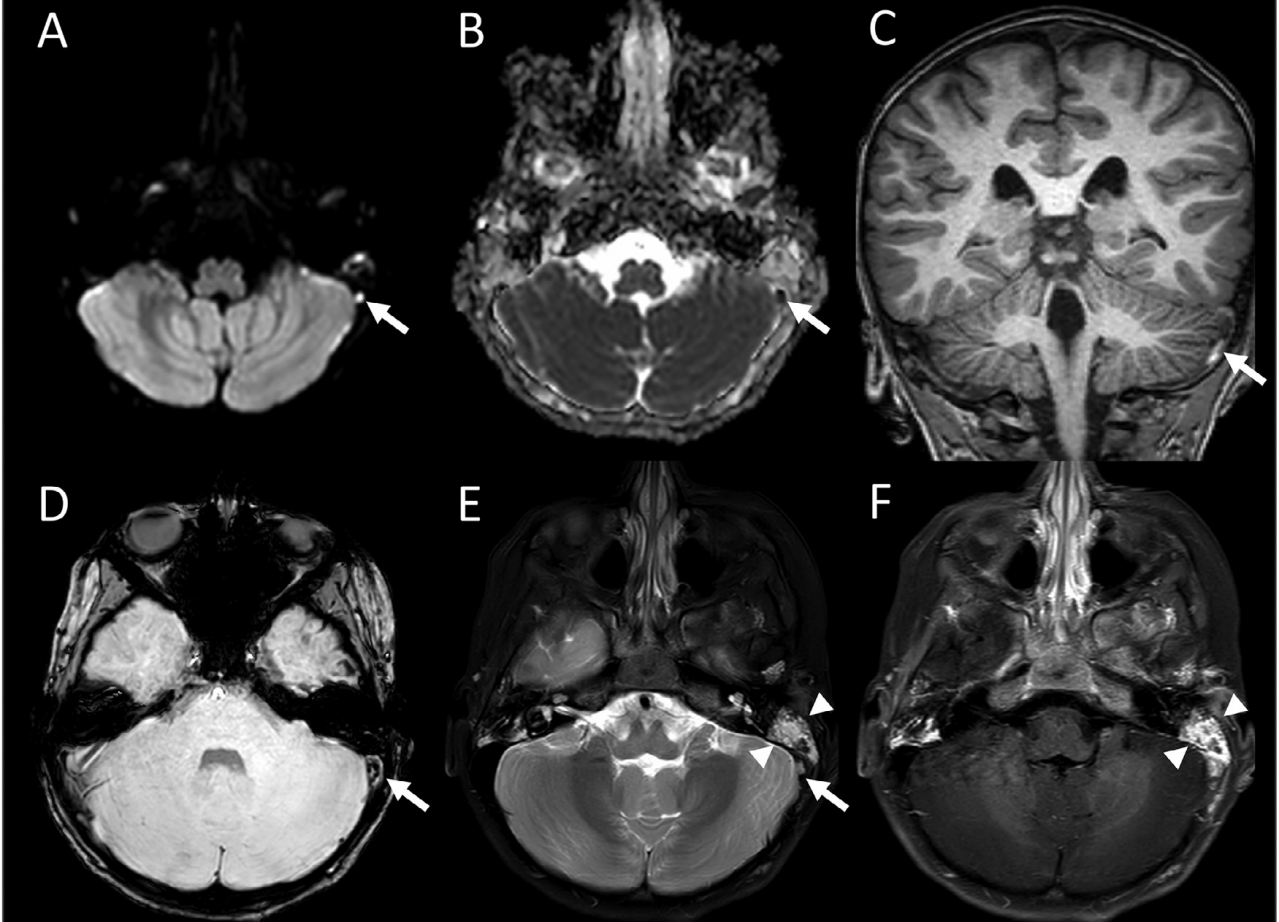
Magnetic resonance imaging (MRI). Axial diffusion-weighted imaging (DWI) (A) and corresponding apparent diffusion coefficient (ADC) map (B) demonstrate a focus of restricted diffusion in the left sigmoid sinus (white arrows), consistent with intraluminal thrombus. Coronal precontrast T1-weighted image (C) demonstrates intrinsic T1 shortening (white arrow) caused by methemoglobin, the oxidized hemoglobin of a subacute thrombus. Axial susceptibility-weighted imaging (D), which is extremely sensitive to iron-containing blood breakdown products, shows a dark signal (“blooming”), indicative of thrombus (arrow). Axial T2-weighted high intensity signal (E) indicates left mastoid fluid (arrowheads). Loss of the normal dark flow void in the adjacent left sigmoid sinus (arrow) indicates thrombosis. Axial postcontrast T1-weighted image shows enhancement (F) caused by mastoid inflammation (arrowheads).
